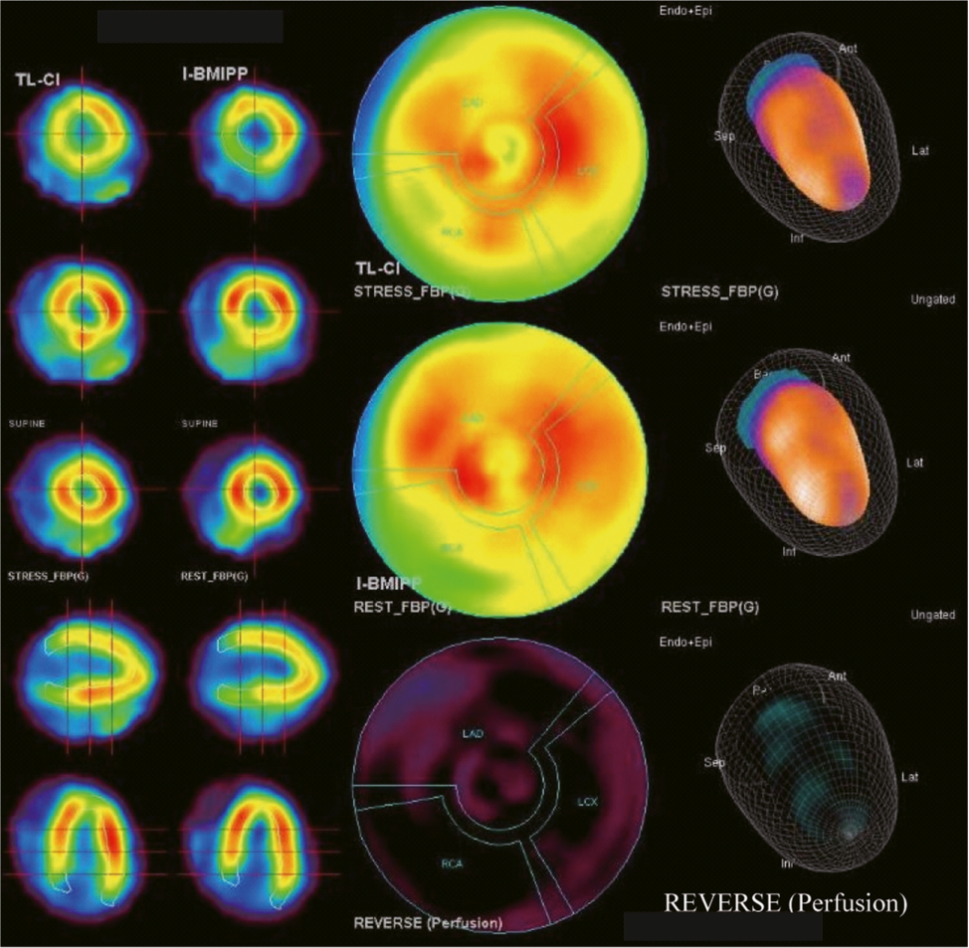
Dual SPECT imaging using Tl-201 and I-123 beta-methyliodophenyl pentadecanoic acid (BMIPP). Widespread distribution of tissue anomalies in the myocardium.
